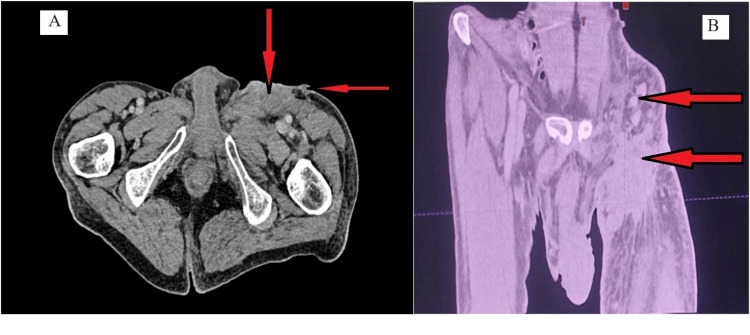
Axial (A) and coronal (B) slice of injected scan CT of the thigh, showing subcutaneous tumor mass on the anterior region of the thigh extending towards the left inguinal region measuring 66 x 30 mm, with the presence of nodular skin lesions (red arrows)
